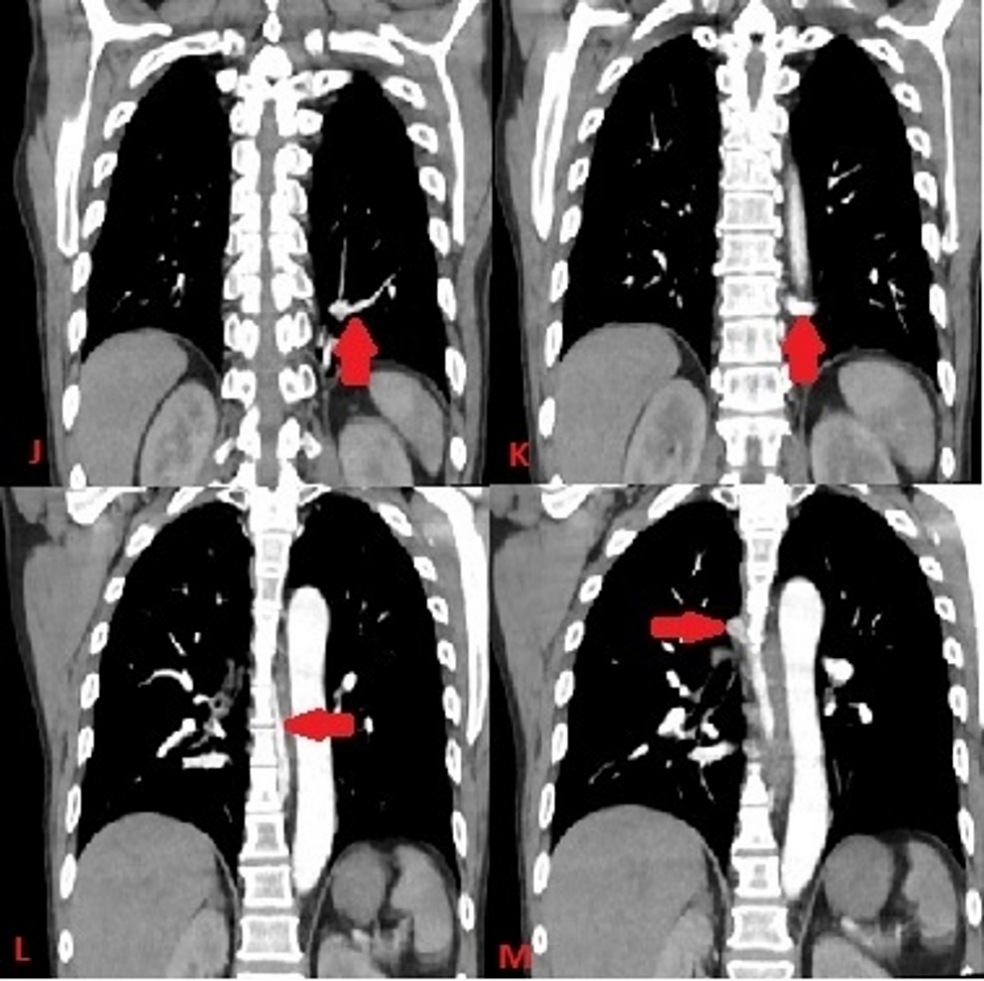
CT of the chest with contrast in the frontal plane and mediastinal window with red arrow demonstrating the course of anomalous pulmonary vein arising from intralobar sequestration (Image J and K) coursing on the side of the aorta (Image L) ultimately joining the azygos vein (Image M)
